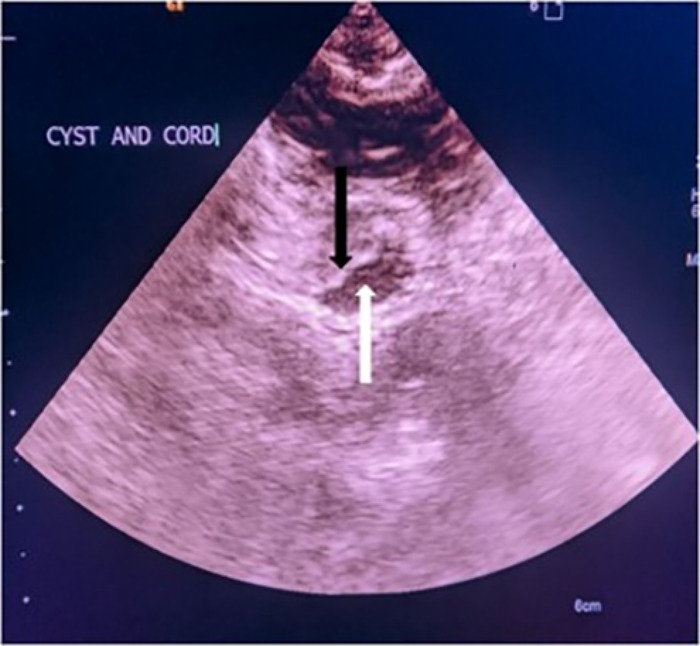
Intraoperative sonogram shows the spinal cord and arachnoid cyst in cross-section. The black arrow indicates the spinal cord, and the white arrow indicates the arachnoid cyst.
